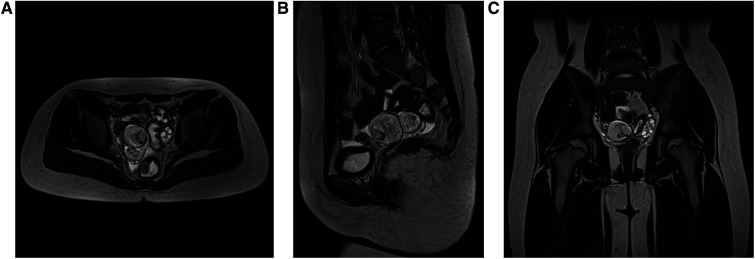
Representative preoperative MRI of patients: (A) horizontal plane, (B) sagittal plane, and (C) coronal plane. MRI indicated that the ovarian tumor was benign. MRI, magnetic resonance imaging.
